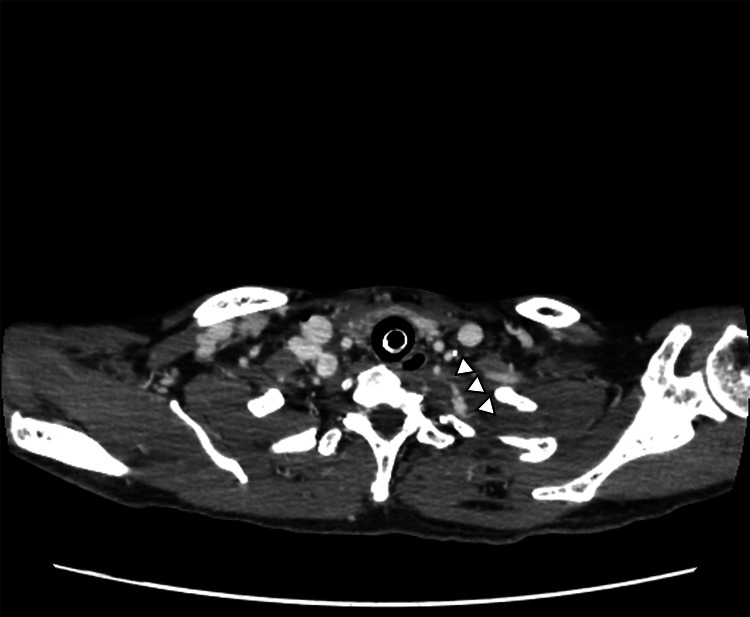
Contrast-enhanced CT Hemothorax on the left chest cavity with extravasation (white arrows) in hematoma on the pulmonary apex region CT: computed tomography
